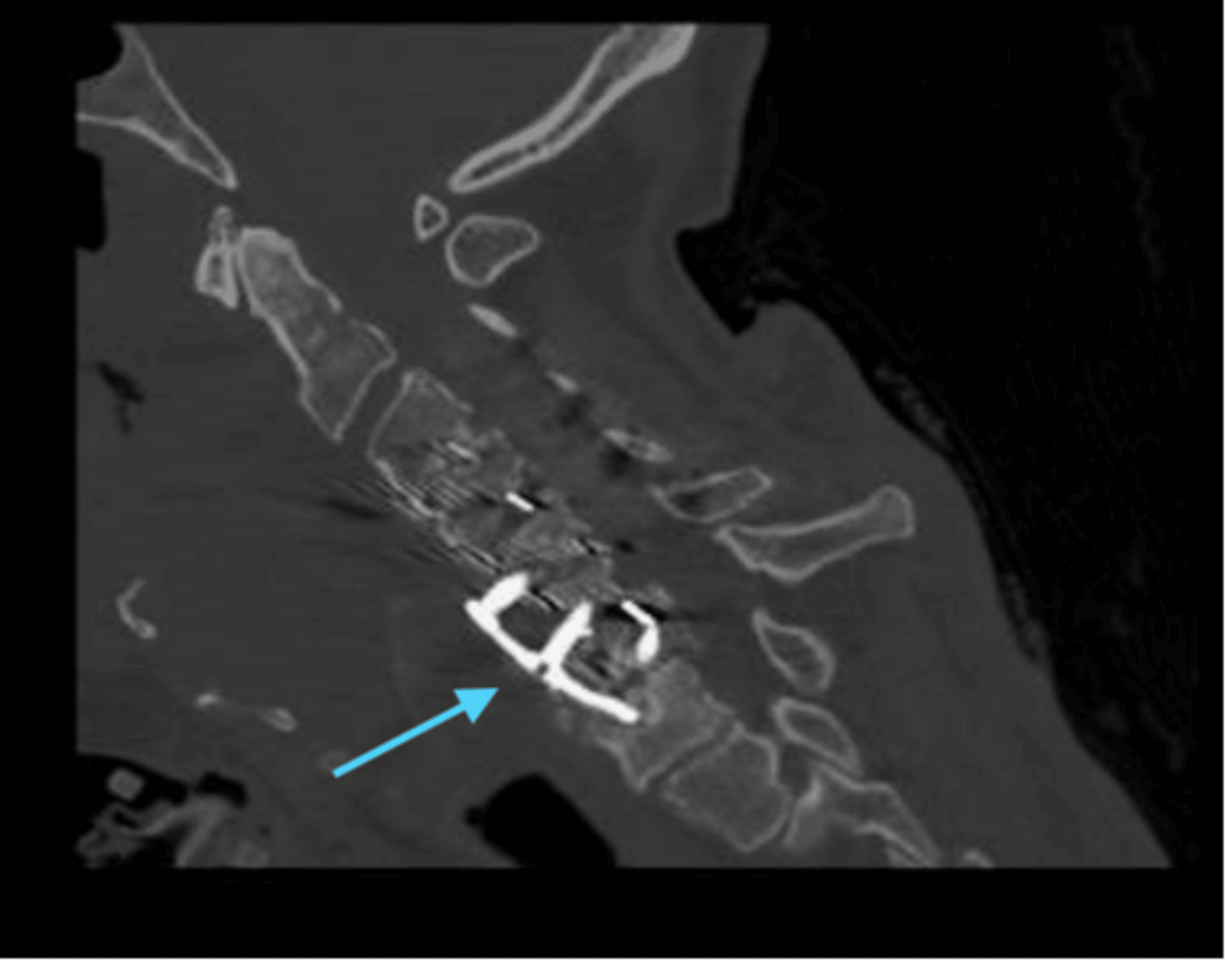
Coronal section obtained from CT of the patient's cervical spine demonstrates new compression fracture deformity of the C7 vertebral body (arrow) CT: computed tomography
